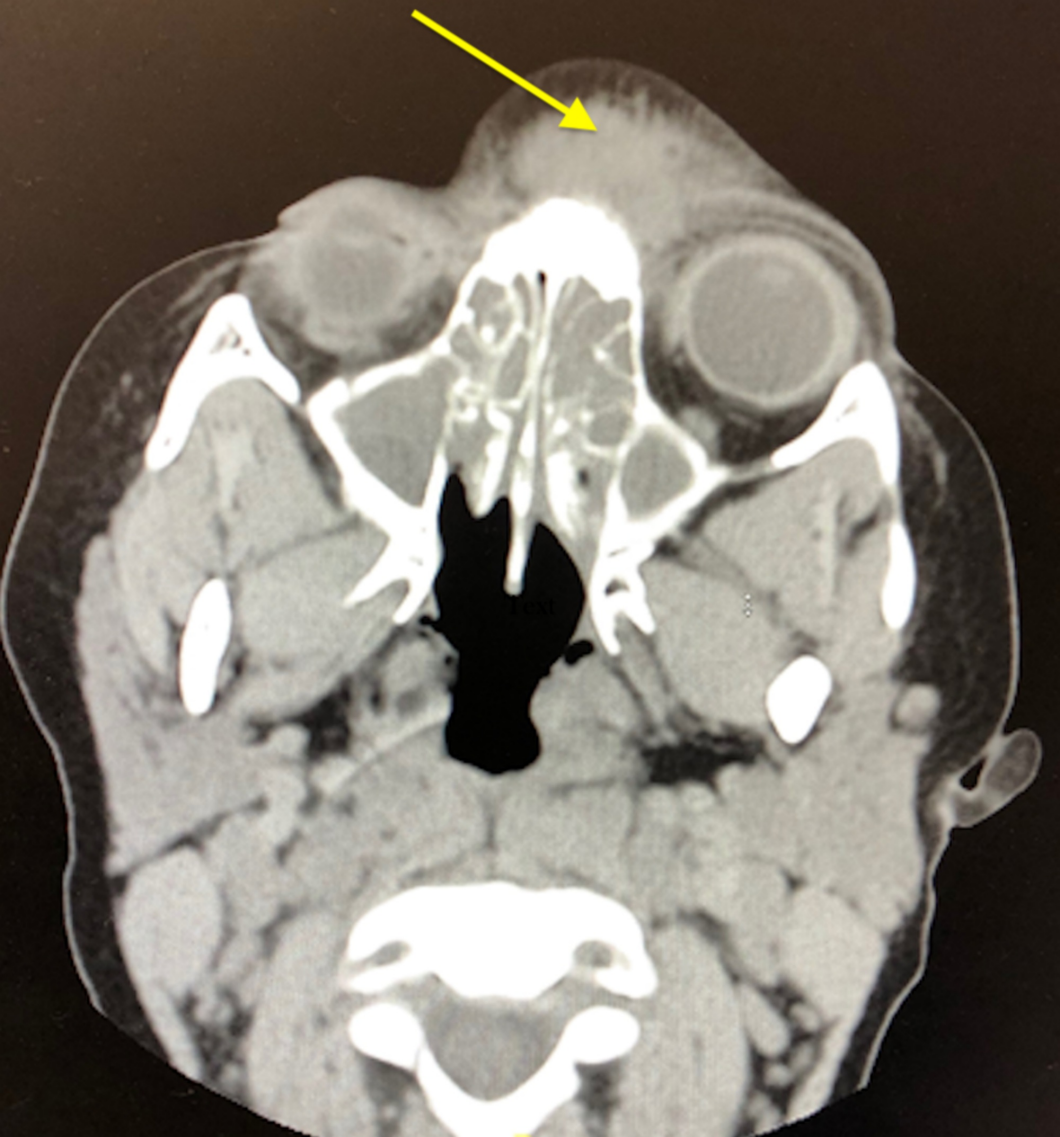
CT scan demonstrating pansinusitis and a large cystic forehead mass (arrow).
